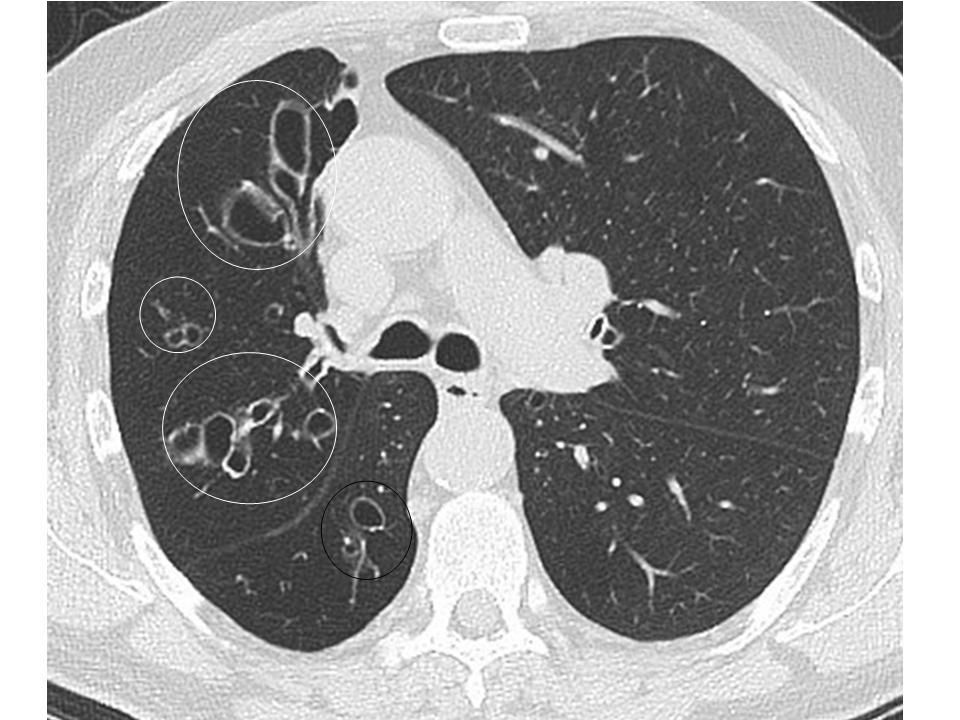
An axial CT image (lung window) of the chest of a 64 years old male with known bronchiectasis shows multiple cluster of cystic areas in the upper (white circles) and lower (black circle) lobes of the right lung. Oblique fissures are well outlined in both the lungs
